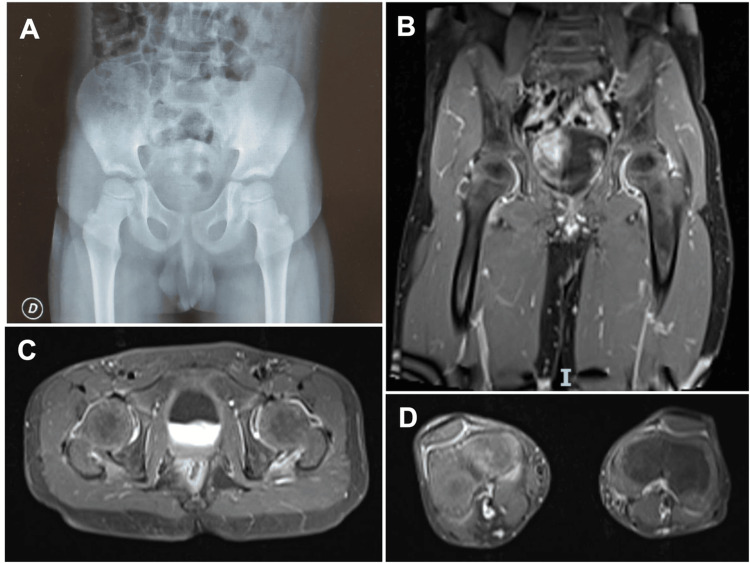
Imaging findings of the hip and knee joints A. Plain radiograph of the pelvis (anteroposterior view) showing no evident erosions or abnormalities in the hip joints. B, C. Coronal and axial MRI images of the pelvis demonstrating mild bilateral joint effusion and synovial enhancement in the hip joints, consistent with arthritis. D. Axial MRI of the knees showing synovial enhancement without evidence of joint effusion.
